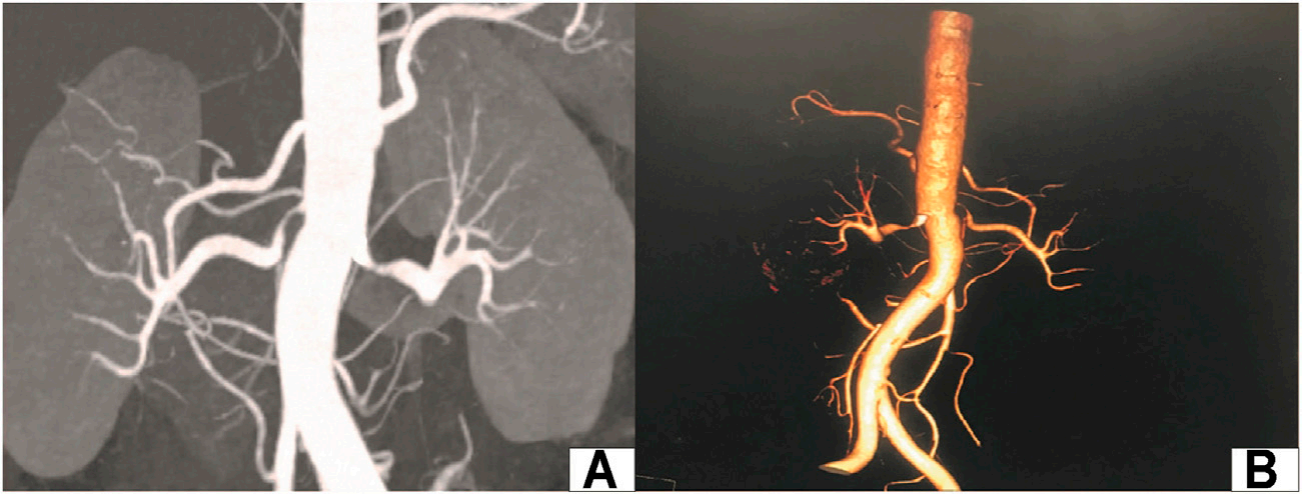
Preoperative images of the renal artery. (A): CT renal angiography; (B): CT 3D reconstruction of renal vessels.
